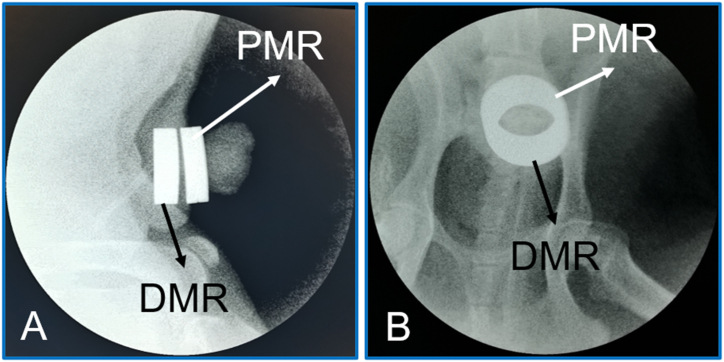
X-ray shows that the daughter and parent magnet rings are positioned as designed. A Lateral X-ray. B Anteroposterior X-ray.
